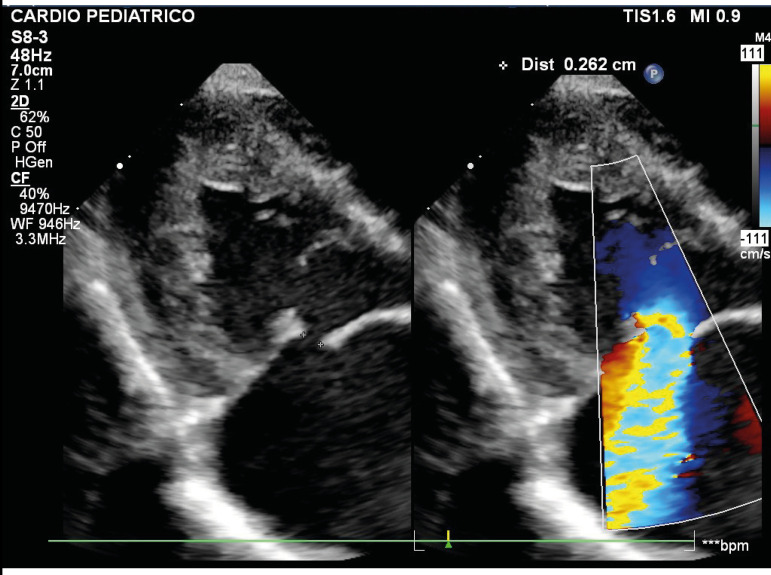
Cross-sectional echocardiography showing a perforation in the anterior leaflet of the mitral valve
